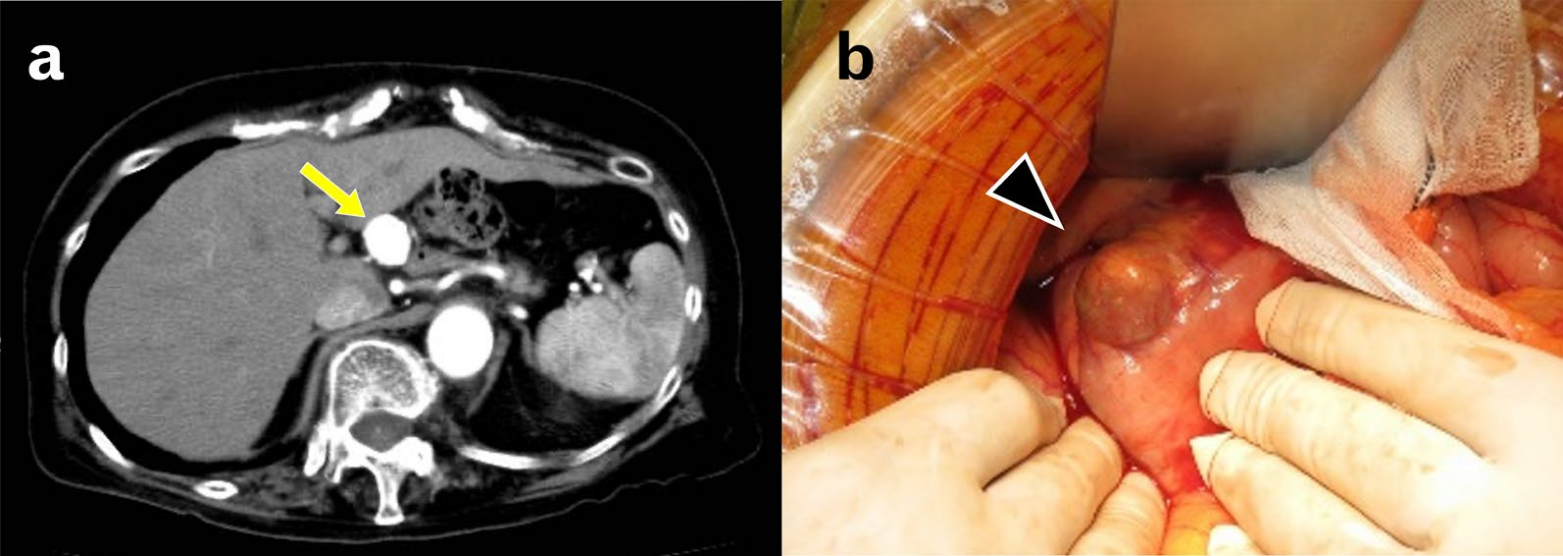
Preoperative contrast-enhanced computed tomography and intraoperative image. a 20 × 17 mm superior pancreaticoduodenal artery aneurysm (yellow arrow). b Intraoperative finding of a superior pancreaticoduodenal artery aneurysm (black arrowhead)
